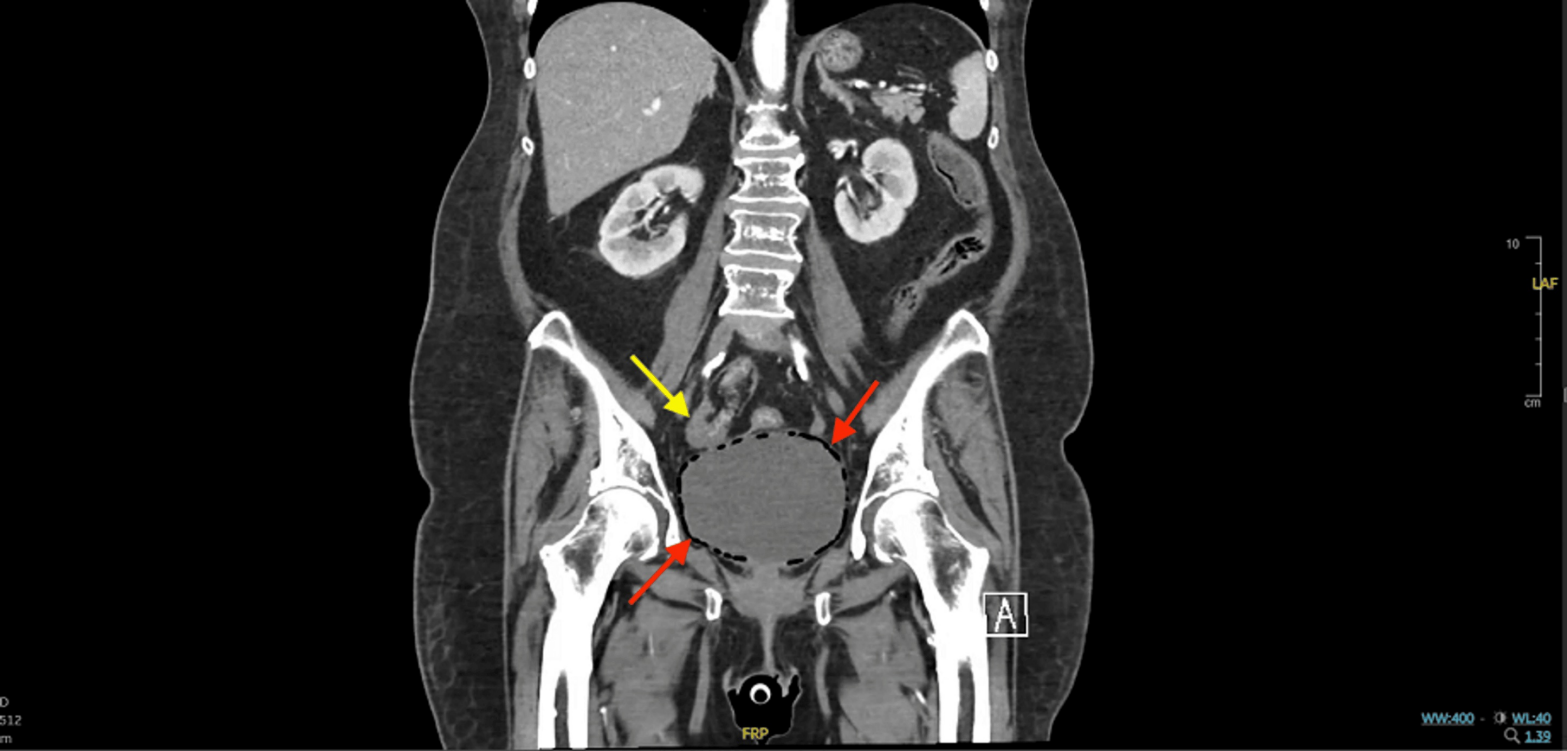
Coronal CT angiography – emphysematous bladder wall gas. Coronal CT angiography of the abdomen and pelvis demonstrating diffuse gas within the bladder wall (red arrows) without extension into the renal collecting system, thereby ruling out emphysematous pyelonephritis. The right ovary (yellow arrow) lies adjacent to the bladder wall with no evidence of fistula, abscess, or necrosis.
